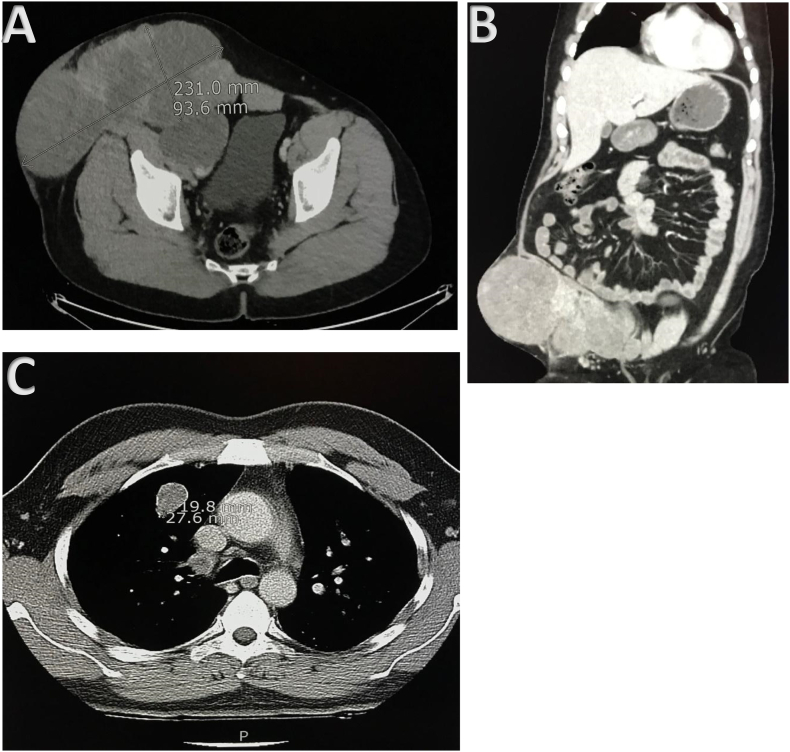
(A-C) CT scans from the most recent presentation (May 2022) in axial (A) and coronal (B) views, respectively. Gross progression of the disease can be seen with regional lymphadenopathy and a conglomerate mass. Also, CT scans in axial view (C) demonstrating the progression of metastatic pulmonary deposits, both in size and number.
